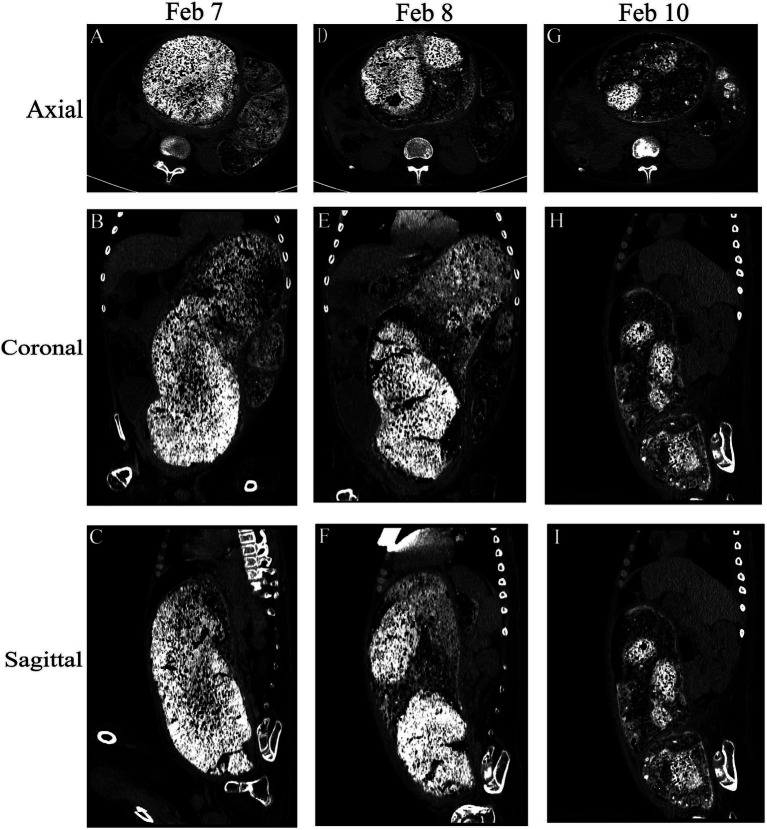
Comparison of the abdominal computed tomography scan before the procedure following multiple enemas. Axial, coronal, and sagittal views were utilized for assessment. (A–C) Initial abdominal computed tomography scan revealed a discernible bowel pattern and a notable abdominal mass measuring 39.5 cm × 18.3 cm × 12.7 cm. (D–F) and (G–I) show the change in fecal characteristics after treatment.
